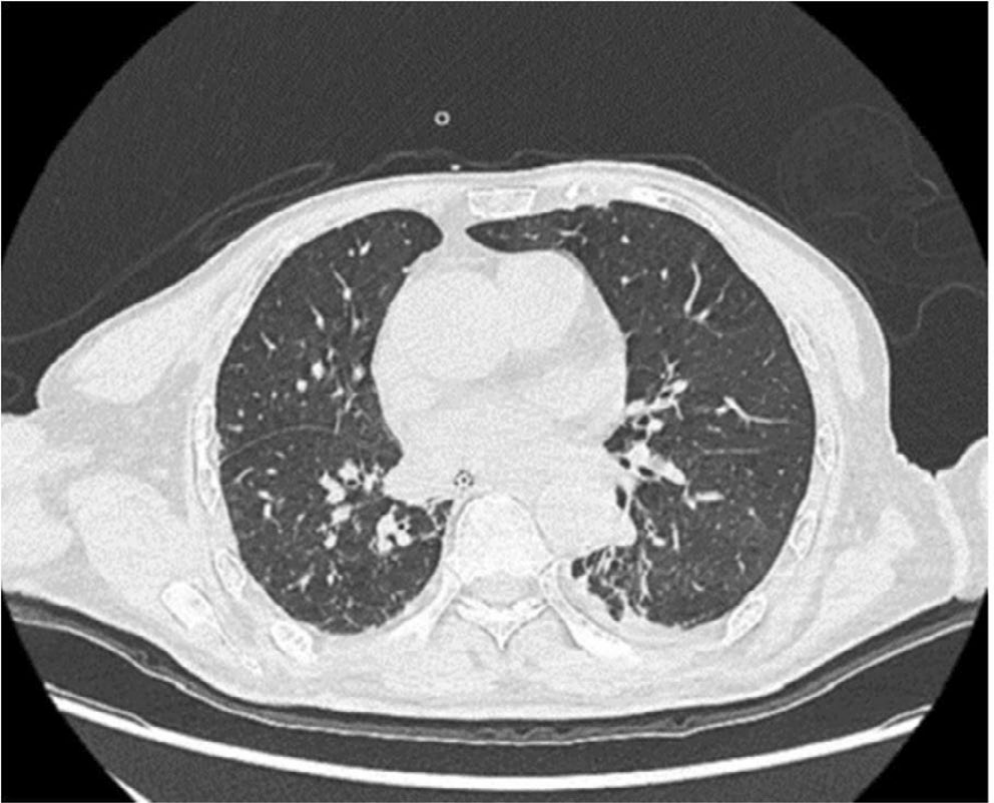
Lung CT showed bronchiectasis and bilateral pneumonia.
